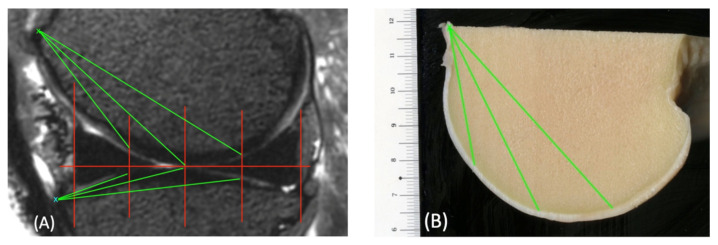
Sagittal view of the medial femorotibial joint in a 3D T2-weighted VIEW sequence (A) and the condyle in a macroscopic scan (B), with marked regions of interest (ROIs, red lines). The base of the condyle (green x) is marked. From this point, the distances to each ROI were measured (green lines). This distance could then be used to find the corresponding ROIs in the macroscopic scans. The same was carried out for the tibia using the tuberositas tibiae (blue x) as the reference point.
